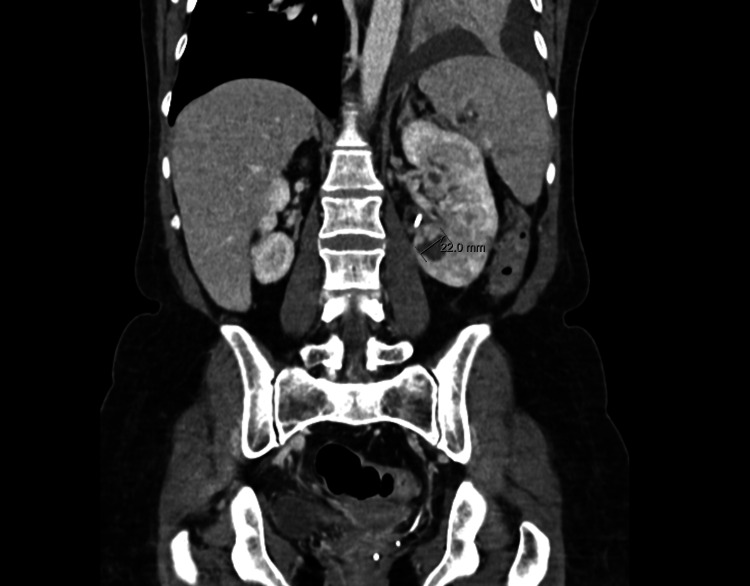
CT showing microabscesses in the left kidney (2022)
